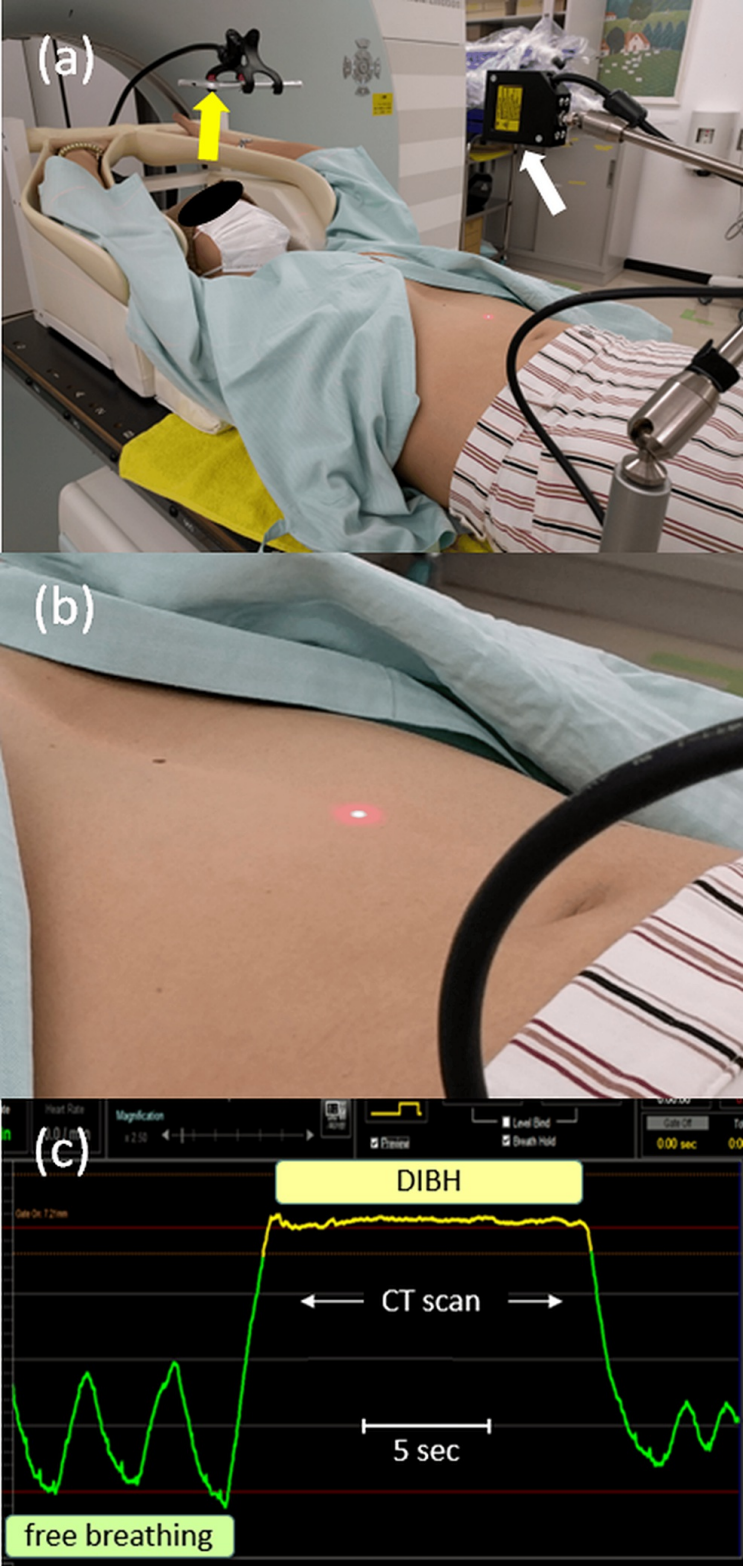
Photographs showing our planning CT scan procedure in deep inspiration breath-hold (DIBH). (a) A laser displacement sensor (white arrow) is attached to the patient couch through an articulated arm, and a compact display (yellow arrow) is placed above the patient’s head for visual feedback of the measured distance to the abdomen during the breath-hold period. (b) A narrow laser beam is projected perpendicular to the patient’s abdomen, visually midway between the xiphoid process and the umbilicus with an aimed laser-abdomen distance of 120 mm. (c) The reflected light is detected on a one-dimensional array sensor, where the detected signal position indicates the distance to the abdomen. Consequently, the free-breathing and the DIBH periods are differentiated by the laser displacement sensor. The planning CT scan was performed during the DIBH period of approximately 10 seconds.
